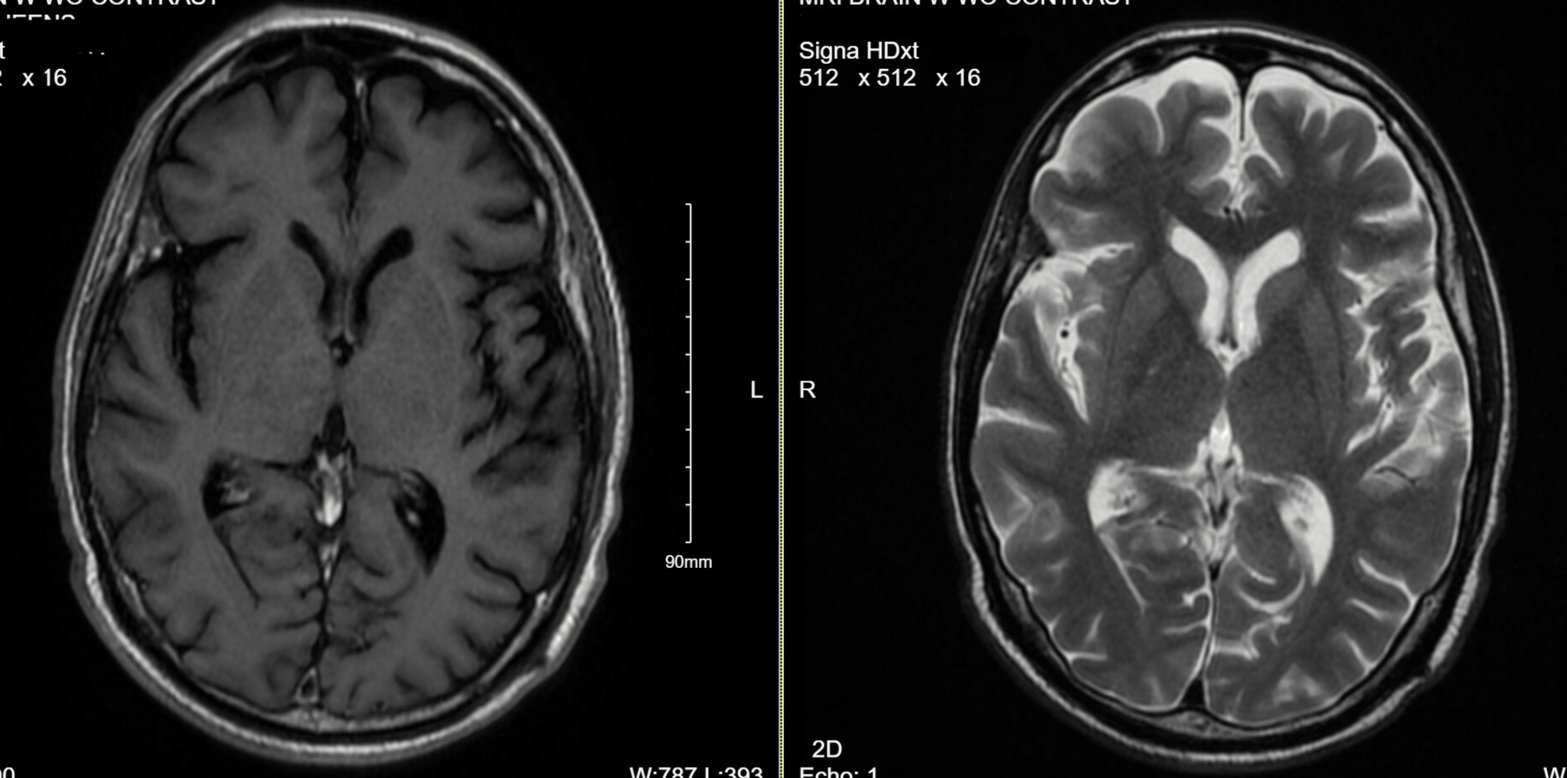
MRI brain - T1-contrast (left-hand pane) and T2 fast spin echo (right-hand pane) images that were negative for leptomeningeal enhancement
